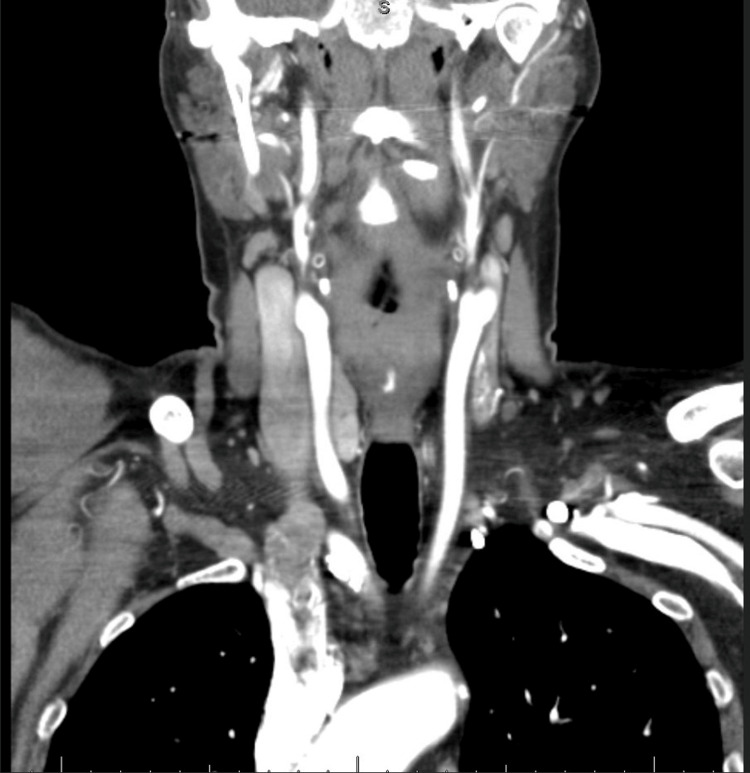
CT angiography of the head and neck demonstrating no evidence of vascular stenosis or aneurysm.
